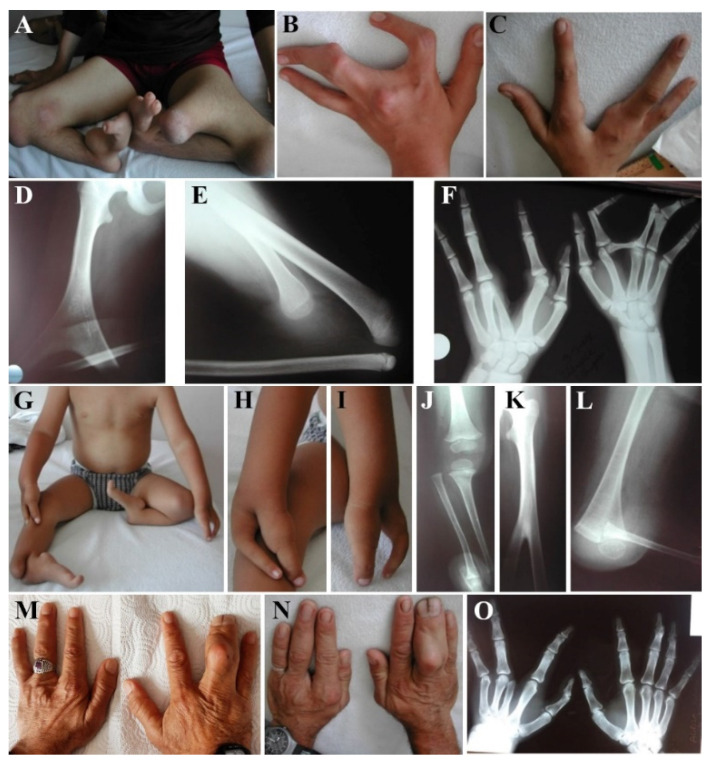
Photographs of the proband (III.3) at age of 13 years: note bilateral femur bifurcation and the fixed clubfoot deformity with only two toes on the right foot and one toe on the left foot (A), and bilateral split hands with the absence of the middle finger (B,C). Radiographs show bilateral bifurcation of the distal portion of the femur and absence of the tibia (D,E). Note five metacarpals bones with complete absence of the phalanges of the third finger on the left hand, and absence of the distal phalanx of the third finger, together with the accessory bone between the second and third metacarpals on the right hand (F). III.7 at the age of 4 years: Femoral bifurcation on the left side with bilateral only one toe on both sides (G) and bilateral split hands with only two digits (H,I). Normal femur and tibia on the right side (J), while femoral bifurcation and absent tibia on the left side are seen on the radiographs (K–L). Hand photographs and radiographs of II.9 (M) and II.8 (N,O): mild split hands with five metacarpal and four phalanges bones together four digits on the left, camptodactyly and syndactyly on the right third and fourth digits.
